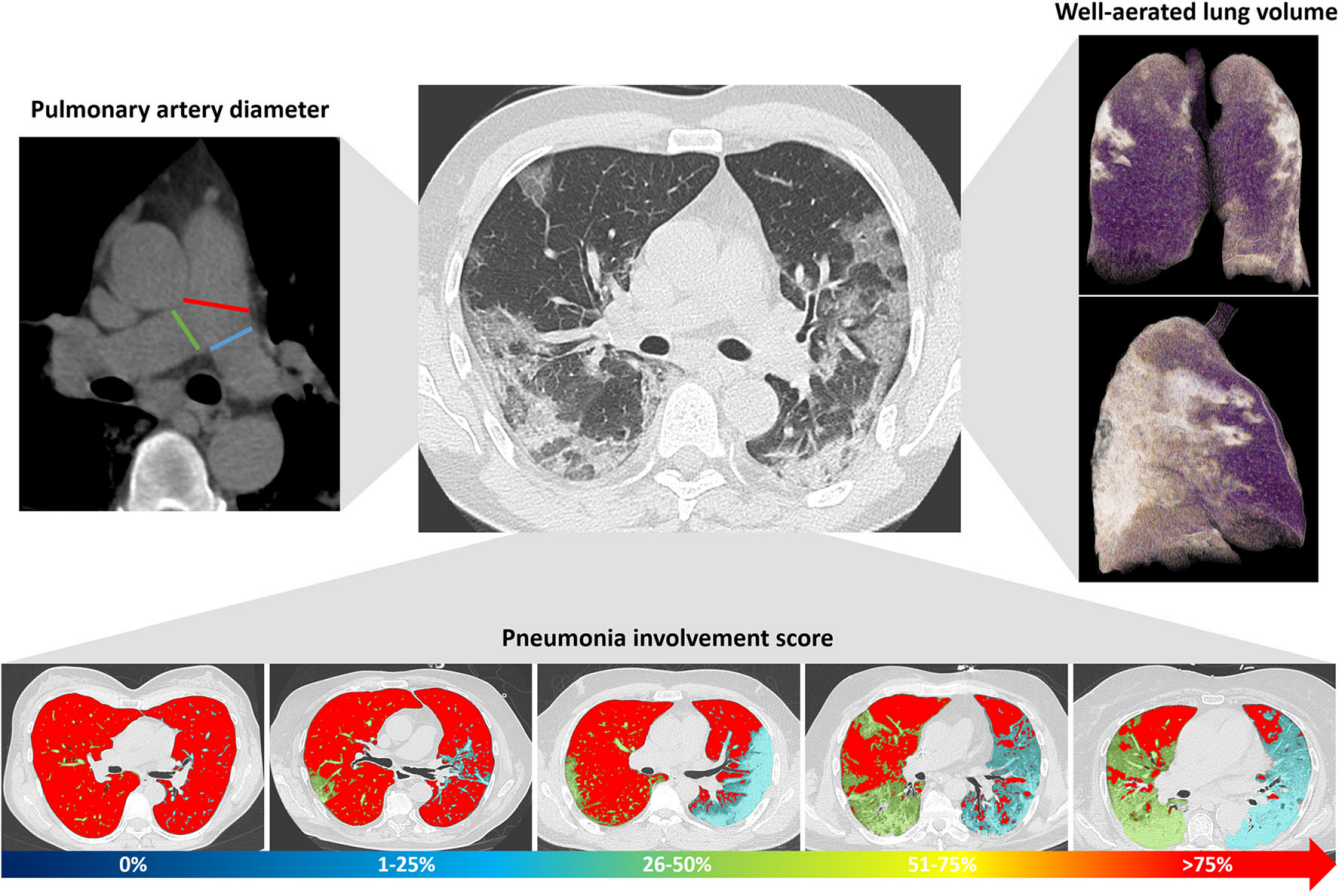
Imaging parameters extracted from chest CT in COVID-19 patients. For each patient, the diameter of the main pulmonary artery (red line) was measured at the level of its bifurcation and the diameter of right and left pulmonary arteries at the level of their origin (green and blue lines, respectively) on the mediastinal window reconstruction (top left of the panel). Using dedicated software, well-aerated lung volume was automatically extracted (violet parenchyma in 3D lung volume rendering on the top right of the panel). Pneumonia involvement was scored from 0 to 4 (score 0: 0%; score 1: 1–25%; score 2: 26–50%; score 3: 51–75% score 4: > 75%); well-aerated lung parenchyma is displayed in red, and pneumonia in green for the right lung and in blue for the left lung (bottom of the panel)
